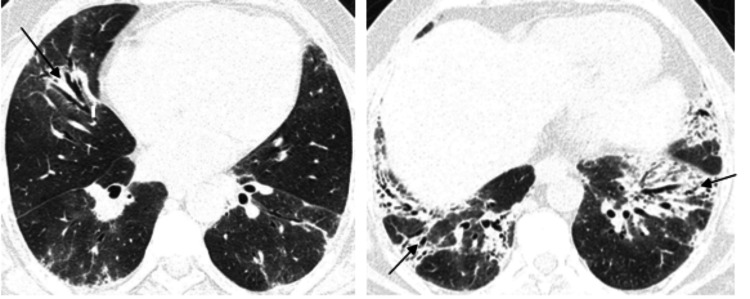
HRCT axial section showing peri-bronchovascular interstitial thickening (arrows) involving bilateral lower lobe, areas of consolidation and air bronchogram.
